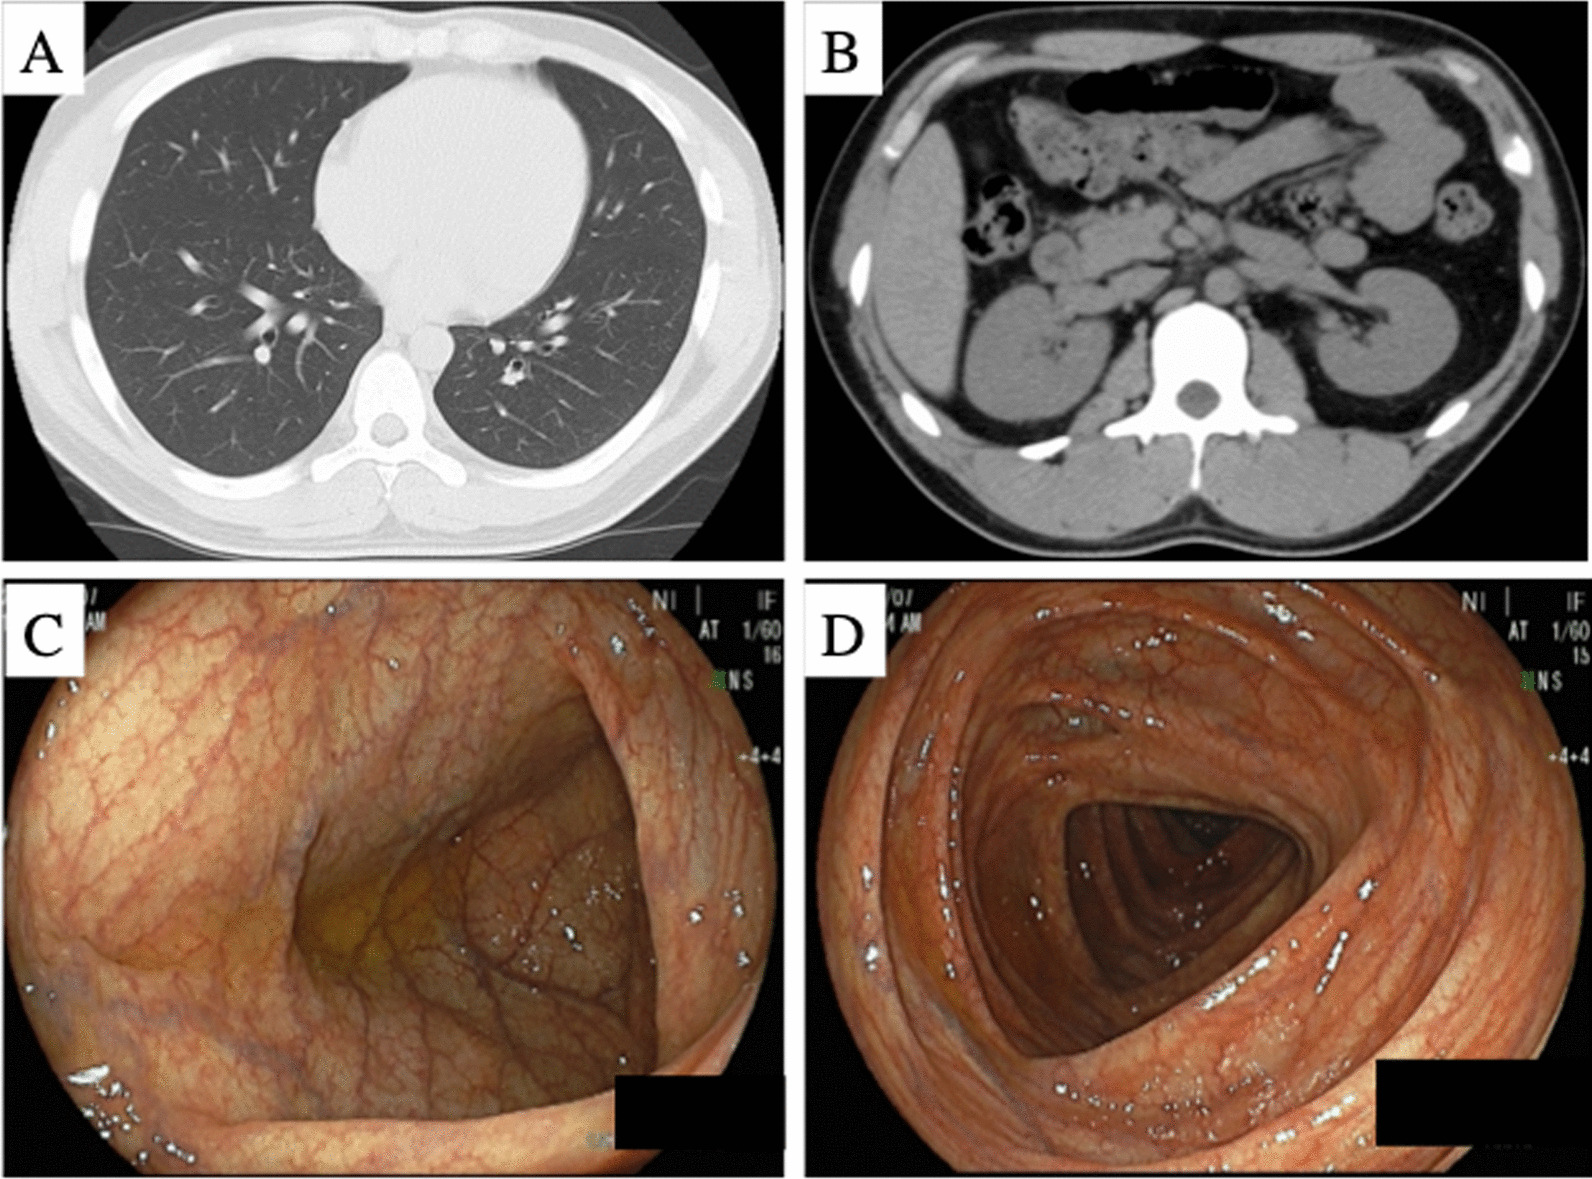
Clinical images of this case: A chest CT scan, B abdominal CT scan, C colonoscopy for sigmoid colon, and D colonoscopy for ascending colon
